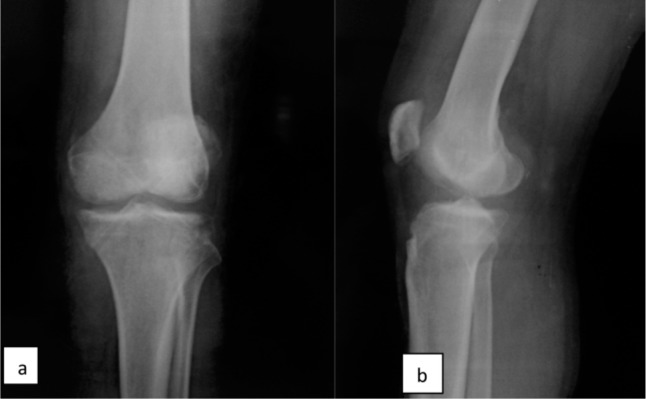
(a) Antero-posterior and (b) lateral radiographs of knee joint showing reduction of joint after removal of Steinman pins.
